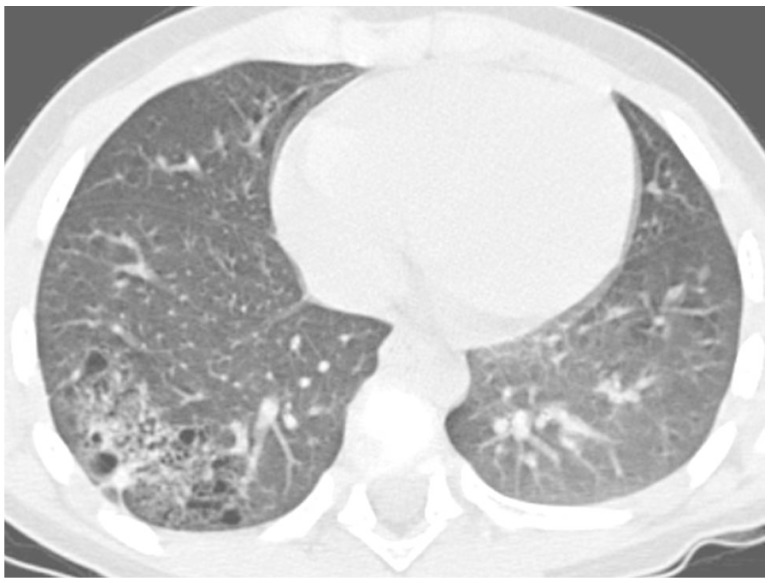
High resolution computed tomography scan of a 6-year-old boy with recurrent pneumonia in the right lower lobe due to congenital cystic adenomatoid malformation (diagnosis confirmed at lung biopsy after lobectomy).
